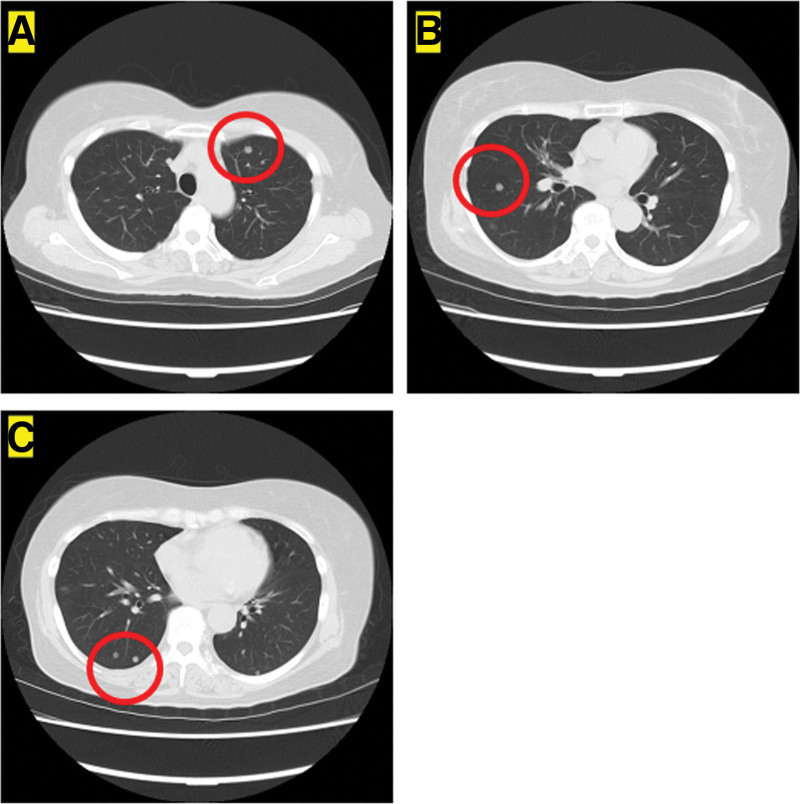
Axial CT image of the lung (A–C). Multiple metastases are observed (A–C). The red circle indicates the metastasis region.
